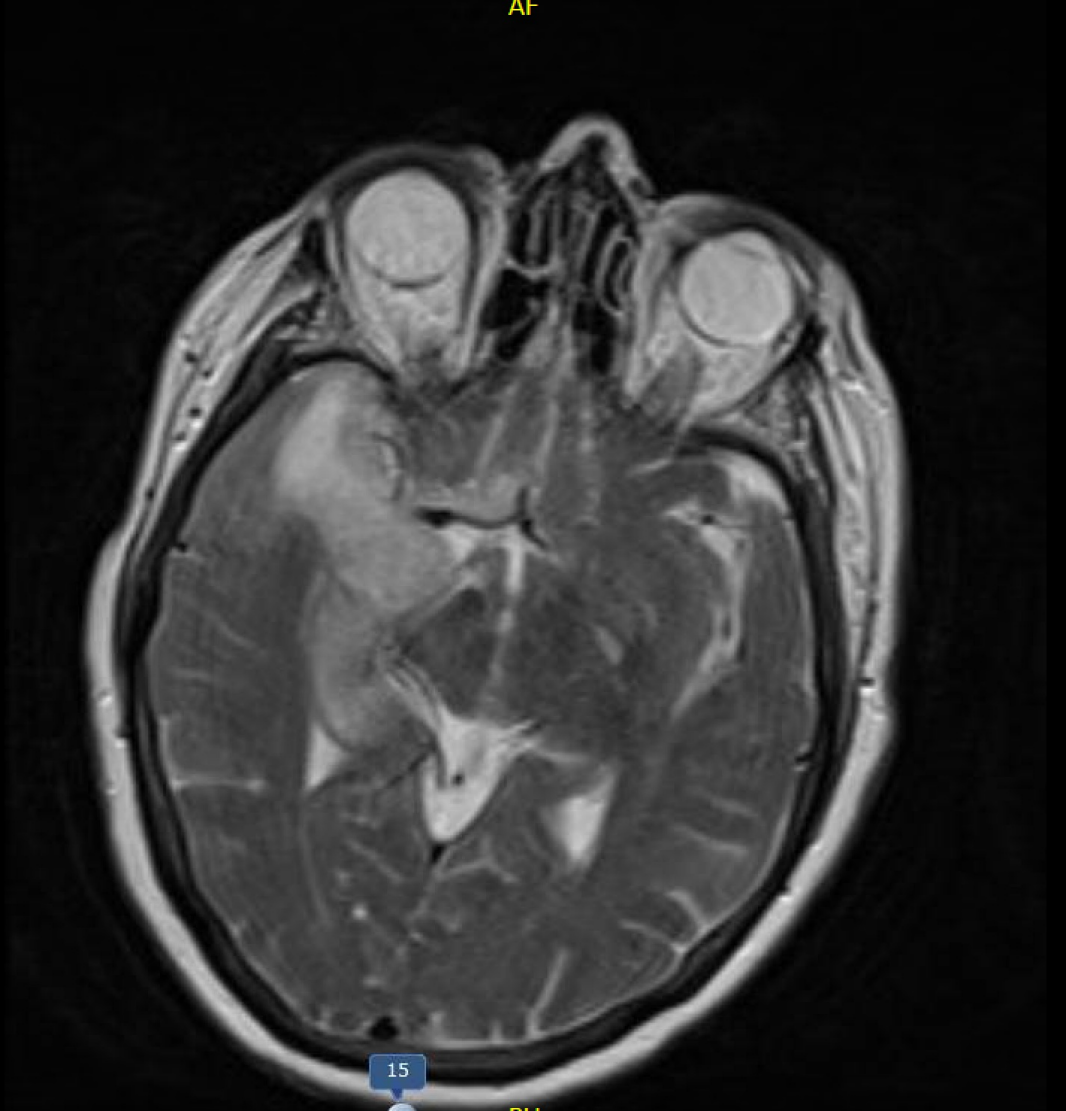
Magnetic resonance imaging (MRI) of the head MRI of the head shows an abnormality of the right temporal lobe, insular cortex, and external capsule regions with a left midline shift of 4 mm
